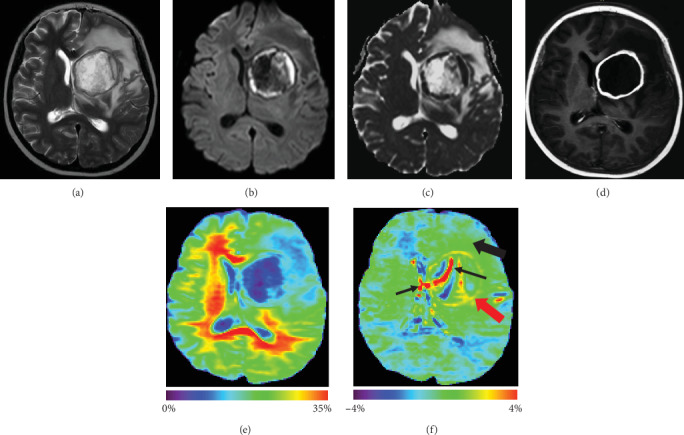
APTw, MTR, and conventional MR images for a 10-year-old girl with pyogenic abscess. T2w image (a) shows a round lesion with surrounding edema in the left basal ganglia region. DWI (b) and ADC map (c) show restricted diffusion in part of the lesion. Gd-T1w image (d) demonstrates an enhancing rim with a nonenhancing necrotic center. The MTR (e) signals are low in the gadolinium-enhancing rim of the lesion and perifocal edema. The APTw (f) signal is high in the gadolinium-enhancing rim of the lesion (red arrow), compared to the perifocal edematous area (black arrow) and CNABT. Note the alternating APTw hyper- or hypointensity in the liquefactive necrotic area and ventricles (black thin arrows).
